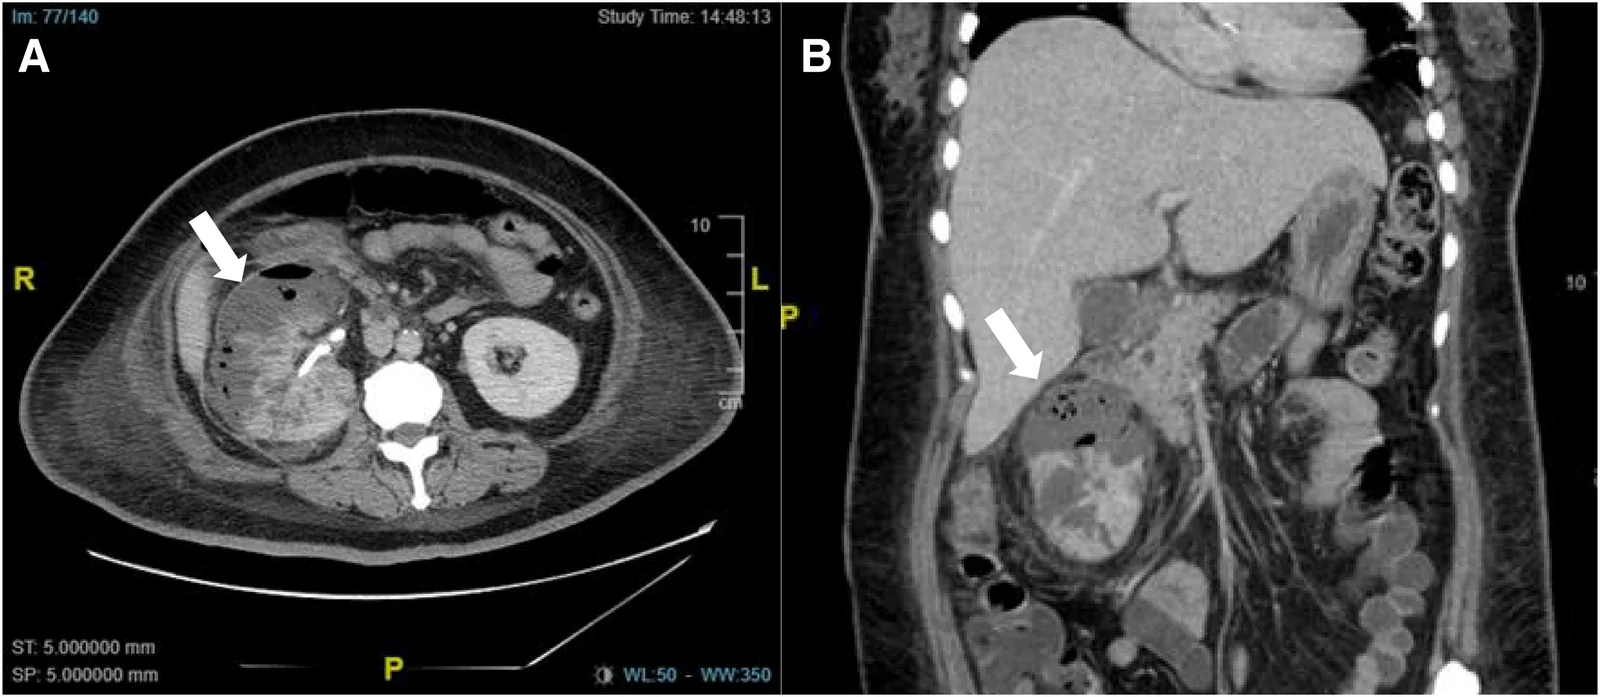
Computed tomography of the right emphysematous pyelonephritis. (A) Axial view showing gas formation within the right renal parenchyma and perinephric space (arrow). (B) Coronal view confirming emphysematous changes in the right kidney with perinephric fluid collection.
